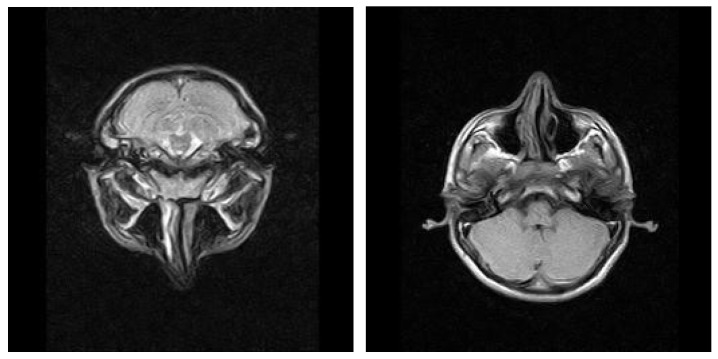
Axial T2-weighted and T1-weighted MRI T2-weighted and T1-weighted MRI axial sections show hypersignal T2-weighted area, isosignal with the T1-weighted brain substance, located in the right middle ear; also, heterogeneous signal area is observed in the right mastoid, indicating the presence of superinfected fluid.
